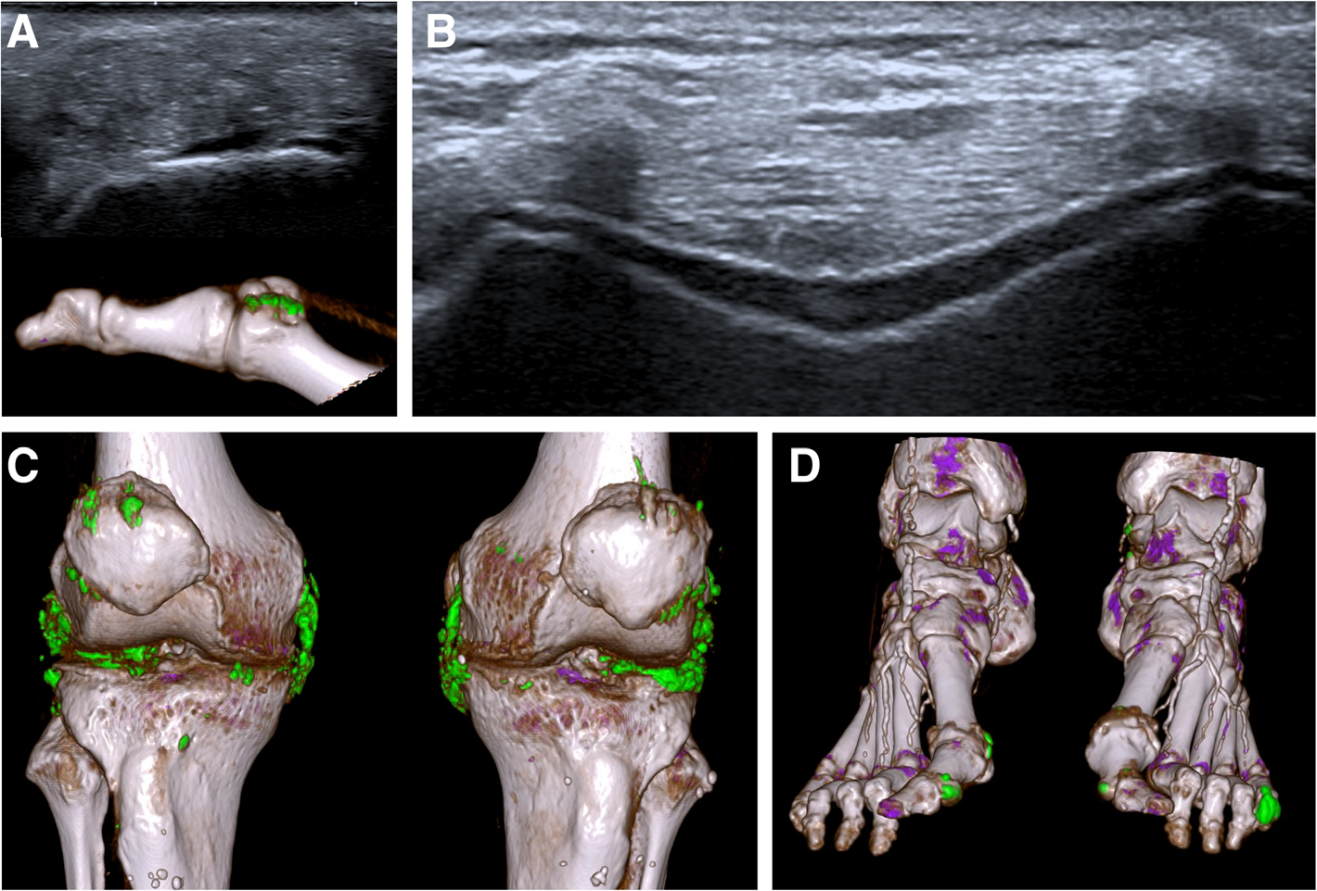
a Tophus of the first metatarsophalangeal joint assessed by ultrasonography (US) (*top*) and dual-energy computed tomography (DECT) (*bottom*). b US double contour sign. c DECT scans of the knees. d DECT scans of the feet
